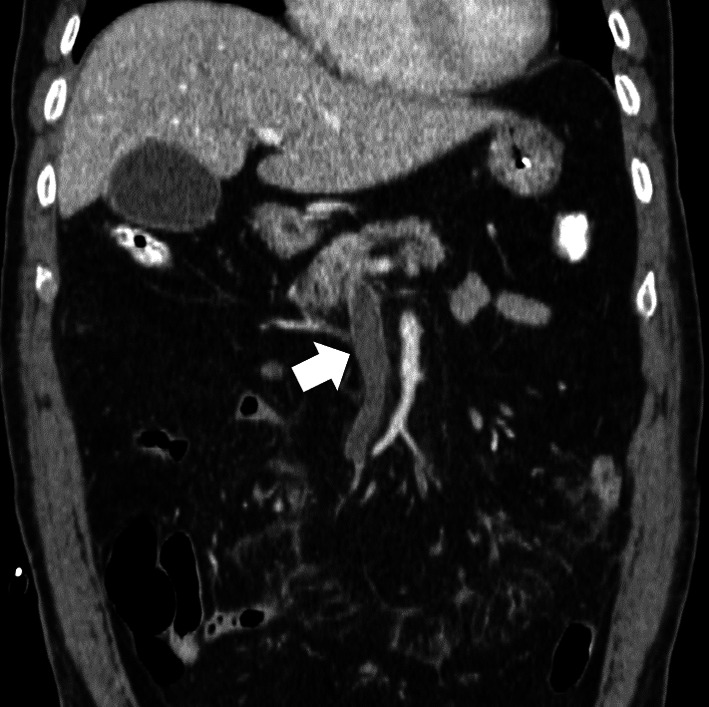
Contrast enhancement computed tomography (CE-CT) findings (coronal view) of Case 1. SMV is completely occluded with a thrombus (arrow). SMV, superior mesenteric vein
